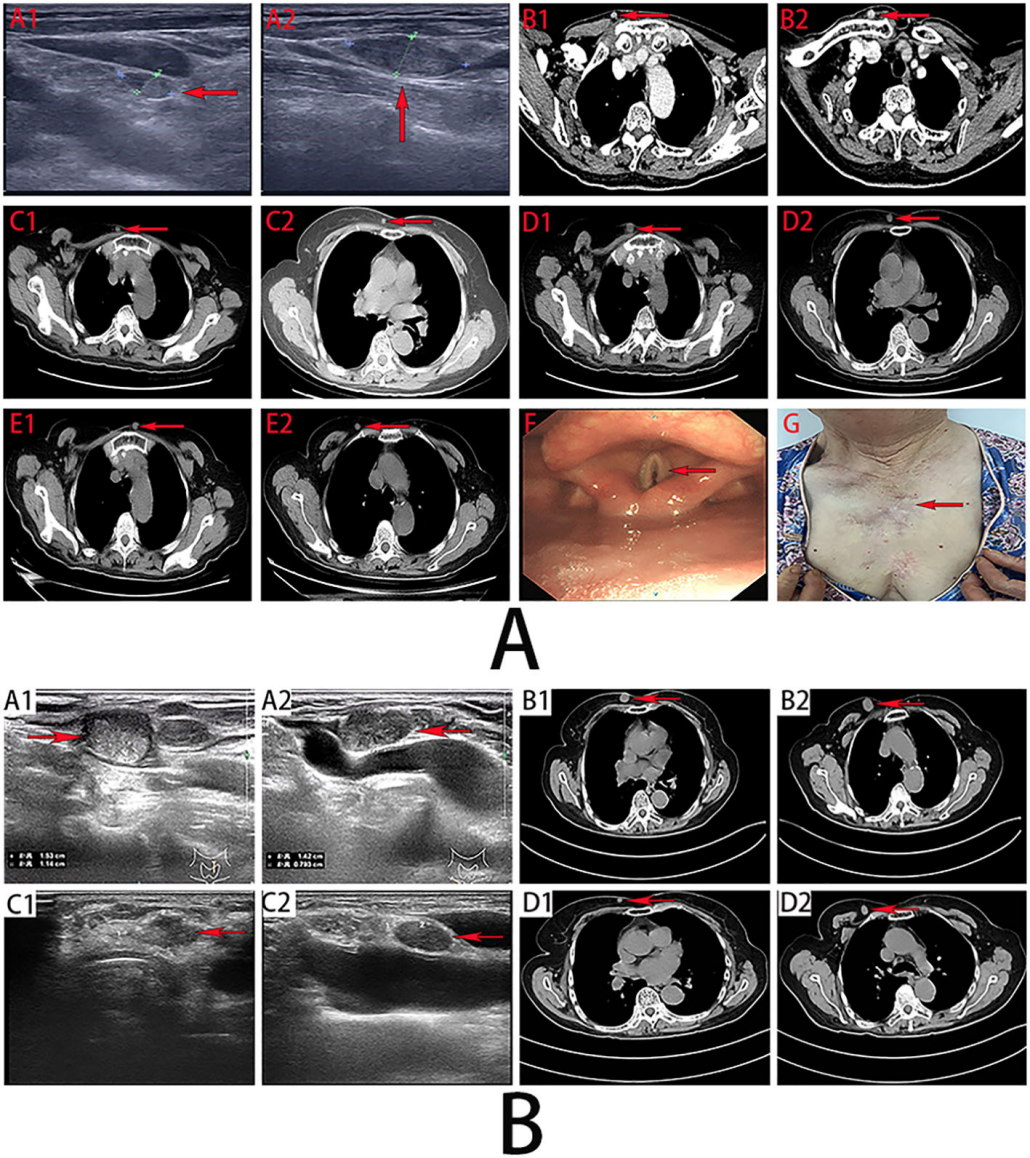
(A). Preoperative imaging diagnosis. (A1, A2) (March 2019): Lymph node metastases of PTC in the right neck (level III) and left neck (level IV). (B1, B2, C1, C2, D1, D2, E1, E2), Chest wall implantations of PTC in November 2020, March 2021, and October 2021, respectively. (F) Laryngoscopy (July 2024) shows poor abduction of bilateral vocal cords. (G) Postoperative presentation of multiple implantation and metastatic lesions on the chest wall and neck. (B). Ultrasonographic images of metastatic lymph nodes in the neck of PTC before and after oral administration of anlotinib (A1, A2 vs C1, C2); CT images of chest wall implantation lesions before and after oral administration of anlotinib (B1, B2 vs D1, D2).
